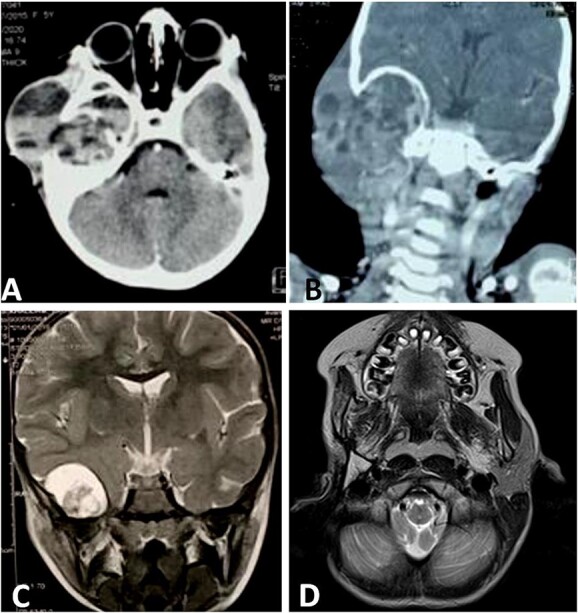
CT scan shows a cystic process of the middle ear cavities with a significant osteolysis of the petrous bone, mastoid and temporal bone (A) with extension to parotid region (B) and homolateral meninge and brain (C). Follow-up MRI shows no sign of recurrences (D).
